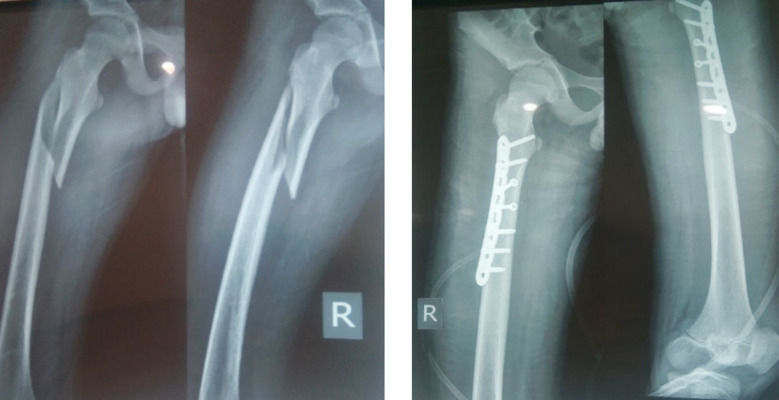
Radiographs-I & II: Pre operative and post operative radiographs of a 9 year old boy.
